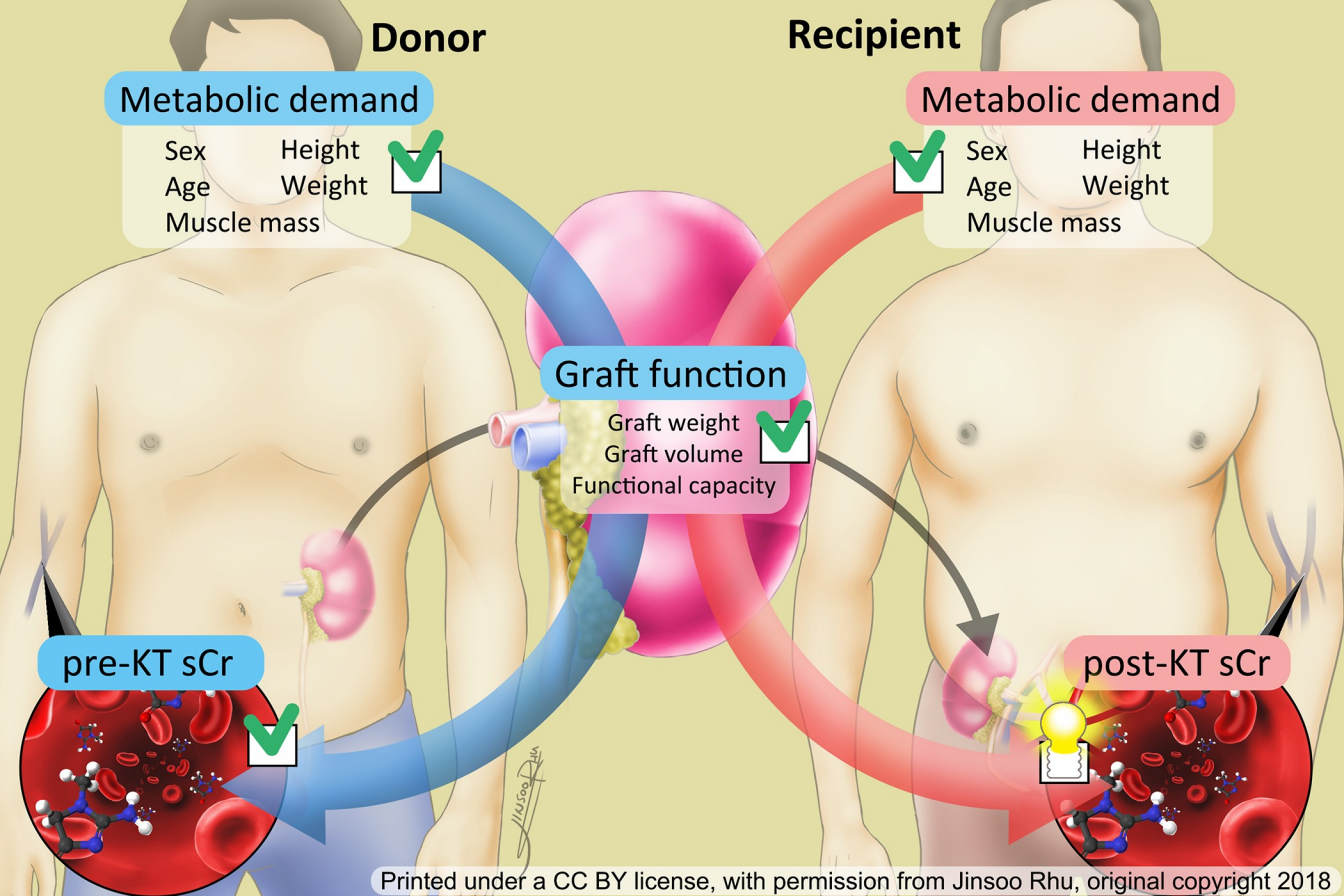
Brief concept of the study hypothesis. The function of the graft kidney includes the graft weight, which represents the nephron mass, and its functional capacity. The graft weight can be measured during transplantation. The volume is calculated using computed tomography. The functional capacity of the graft kidney can be estimated with regard to the metabolic demand of the donor and donor’s pre-transplant serum creatinine. Therefore, the post-transplant serum creatinine level of the recipient can be estimated by replacing the data from the donor’s metabolic demand with that of the recipient. Green check points represent data that can be measured. The post-transplant serum creatinine of the recipient is calculated based on pre-existing data. KT kidney transplantation, sCr serum creatinine.
